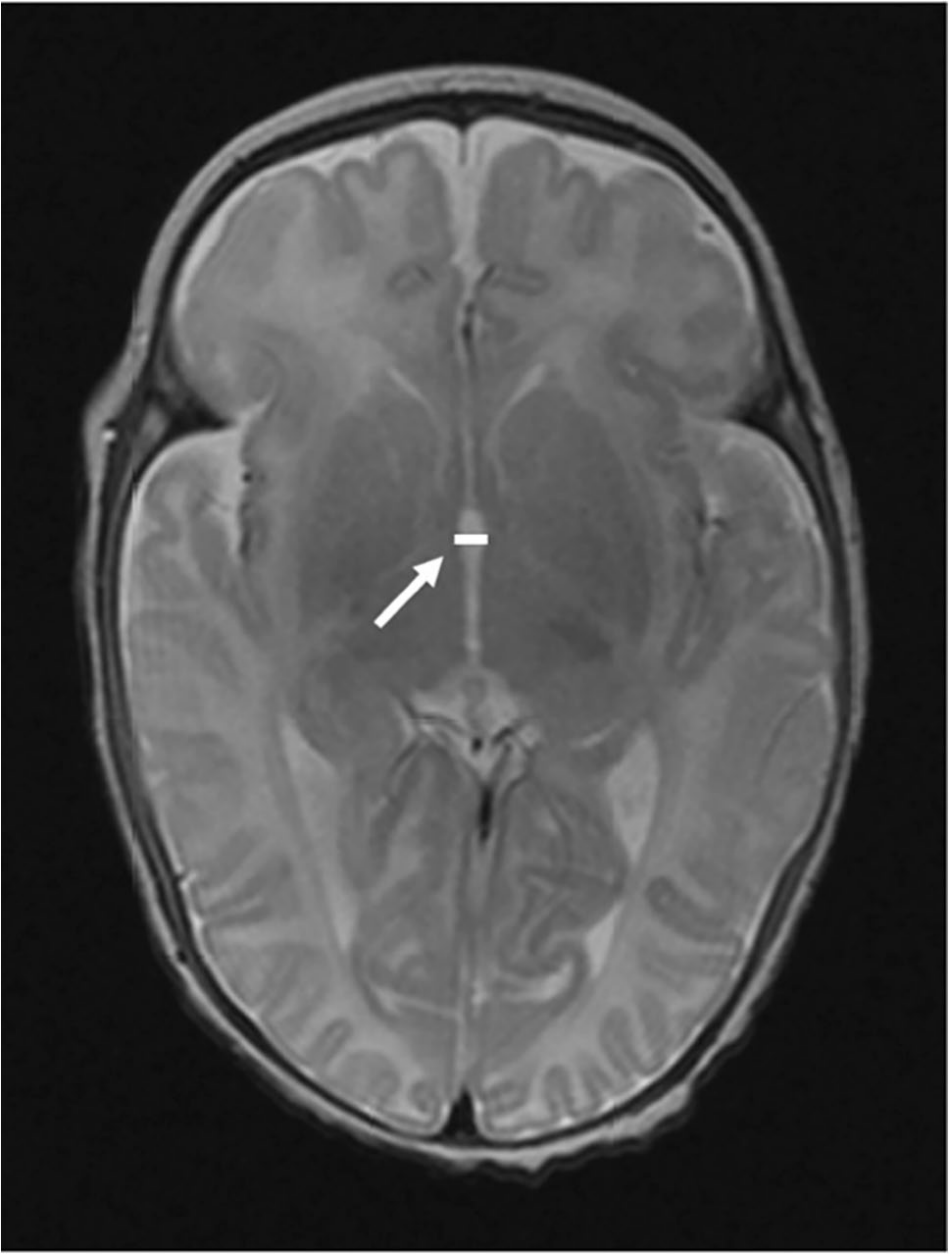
A 16-week-old girl born extremely premature at 25 gestational weeks with magnetic resonance imaging performed at term-equivalent age. Axial T2-weighted image without contrast at the level of the third ventricle shows third ventricle width (*arrow*). The infant had no intracranial haemorrhage or other pathology identified on cranial ultrasound in the neonatal period
